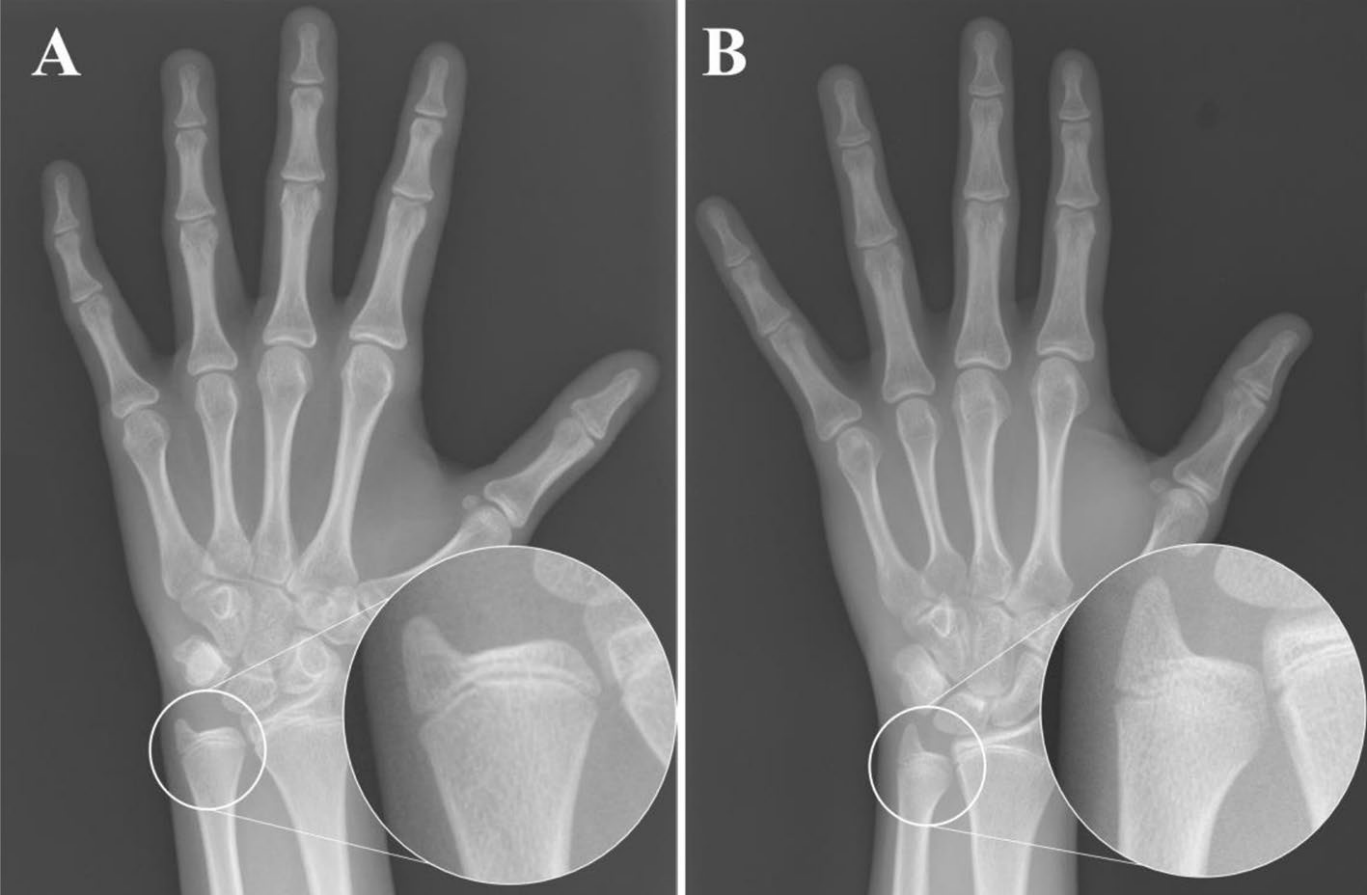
Radiographs of hands classified as Sanders maturation stage (SMS) 7A and 7B. In SMS 7A, all phalangeal physes are fused and only the distal radial physis is open with narrowing of the medial physeal plate of the distal ulna (A). In SMS 7B, there is fusion of > 50% of the medial growth plate of the distal ulna (B)
